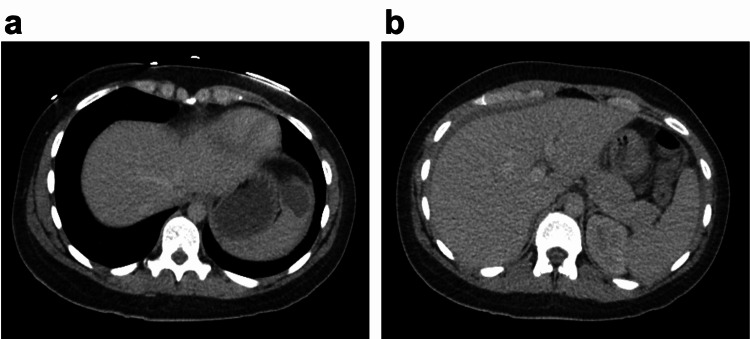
Axial CT images of the splenic cyst (a) and hemoperitoneum (b)
